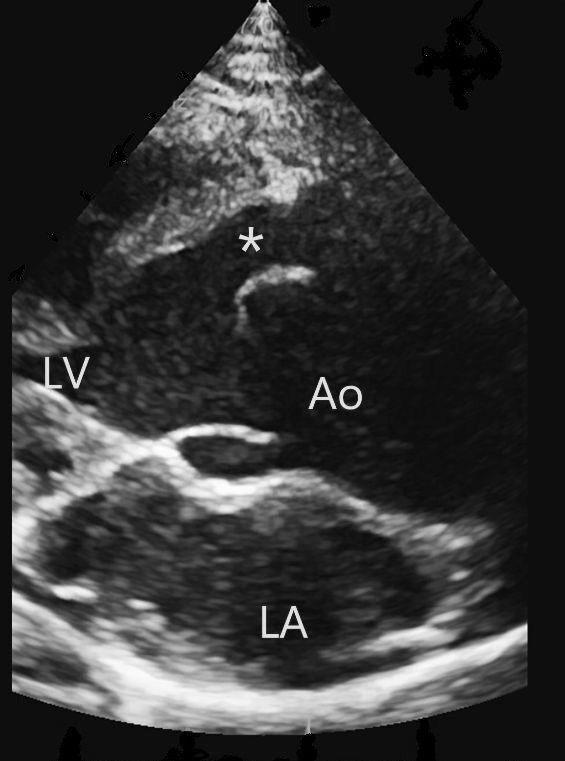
Echocardiographic picture of ALVT. Transthoracic parasternal
